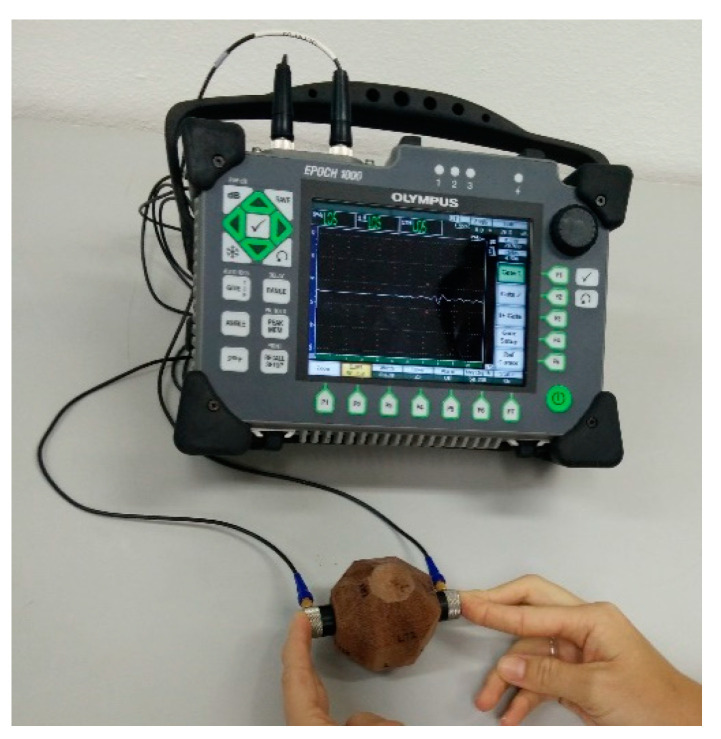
Ultrasound test on polyhedra for wood characterization.
